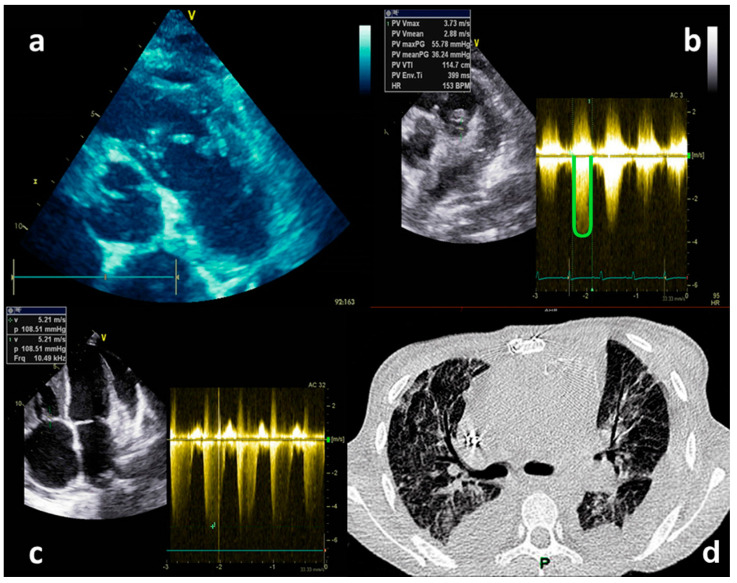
Echocardiography TTE and CT imaging−Case 1. (a) TTE−Multiple floating vegetations in the right ventricular outflow tract, pulmonary valve, and on the wall of the pulmonary artery. (b) TTE−Gradient across pulmonary valve. (c) TTE−Maximal velocity of the tricuspid regurgitation. (d) MSCT−Multiple septic embolizations in the lung parenchyma, lung atelectasis, pleural effusion.
